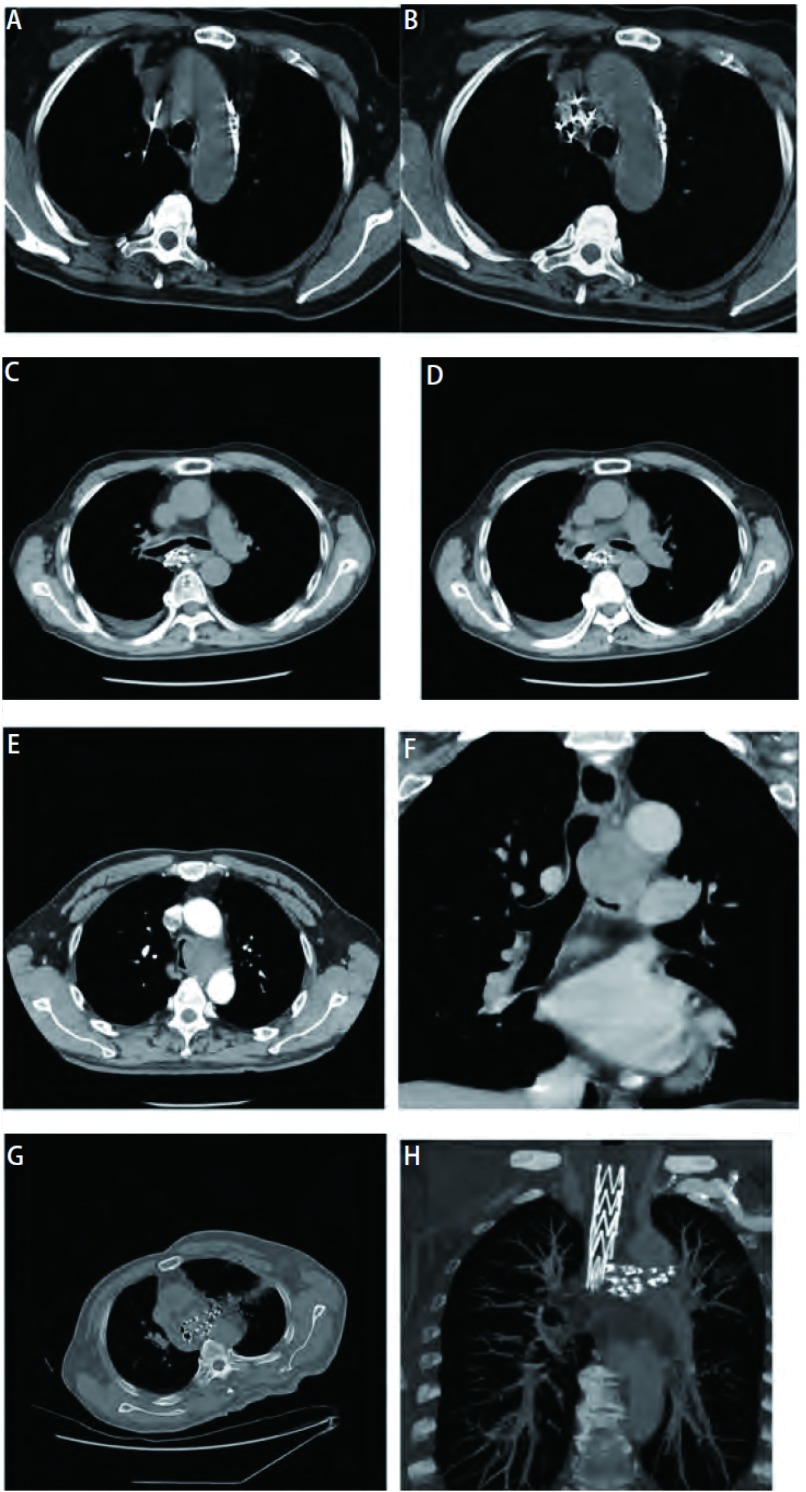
纵隔内恶性肿瘤和淋巴结转移癌。A, B:4R淋巴结转移癌, 穿刺及粒子分布; C, D:7区纵隔型肺鳞癌治疗后粒子分布; E, F:3L、4L纵隔型肺鳞癌治疗前CT增强扫描显示巨大肿瘤; G, H:与E, F同一患者, 治疗后粒子分布。 Mediastinal malignant tumors and lymph node metastases.A, B:The punctue region and particles distribution of mediastinal malignant tumor in 4R region; C, D:Particles distribution of mediastinal lung squamous cell carcinoma in 7 region; E, F:Enhanced CT scanning shows the giant tumor of mediastinal lung squamous cell carcinoma in 3L and 4L region before therapy; G, H:Particles distribution after therapy.
